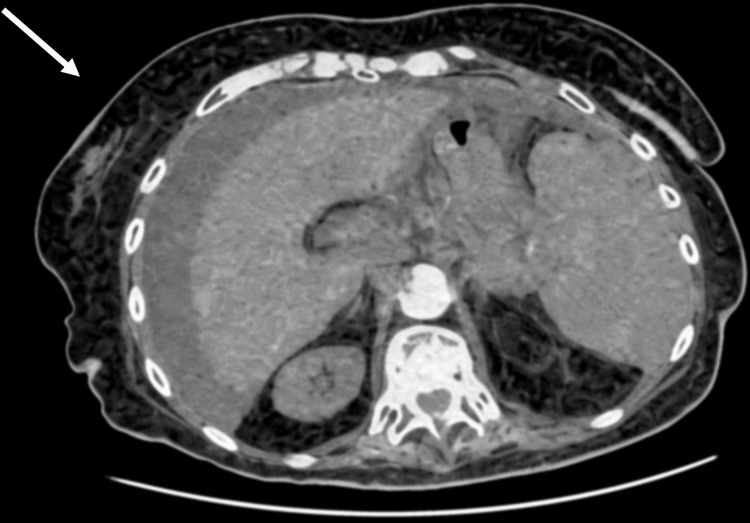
Axial contrast-enhanced CT image demonstrating omental involvement Axial contrast-enhanced CT image demonstrating increased soft tissue density within the anterior abdomen (arrow), suggestive of omental involvement.
